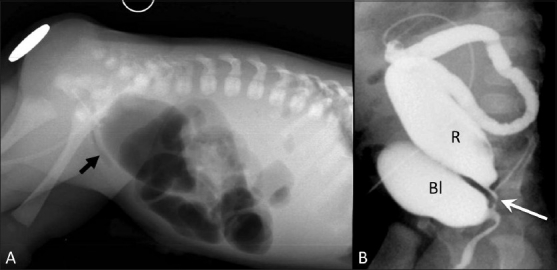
Anorectal malformation: High imperforate anus with recto-uretheral fistula. Cross-table lateral radiograph (A) shows absence of air in the anal area (metallic marker at the anal verge). A lucent curvilinear air shadow is seen anteriorly (arrow) representing air in the urinary bladder as a result of the fistula. Loopogram (B) of the same patient confirms the recto urethral fistula and outlines the rectum and the bladder
